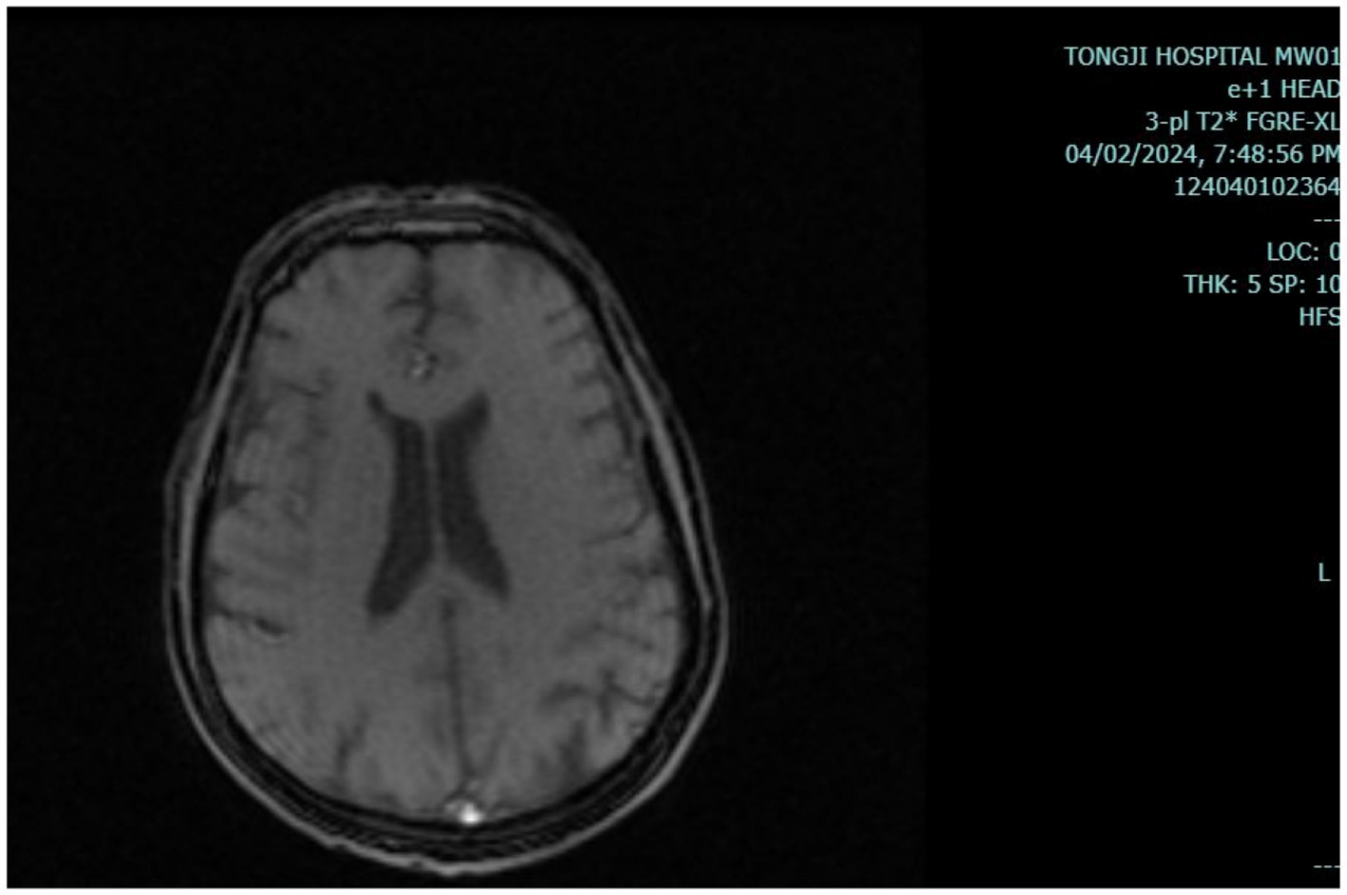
Head MRI. Head magnetic resonance imaging (MRI) shows brain atrophy.
